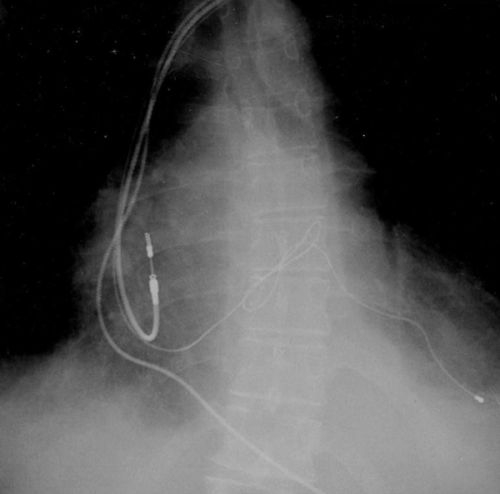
X-ray chest AP view showing 'granny knot' of left ventricular lead in coronary sinus
